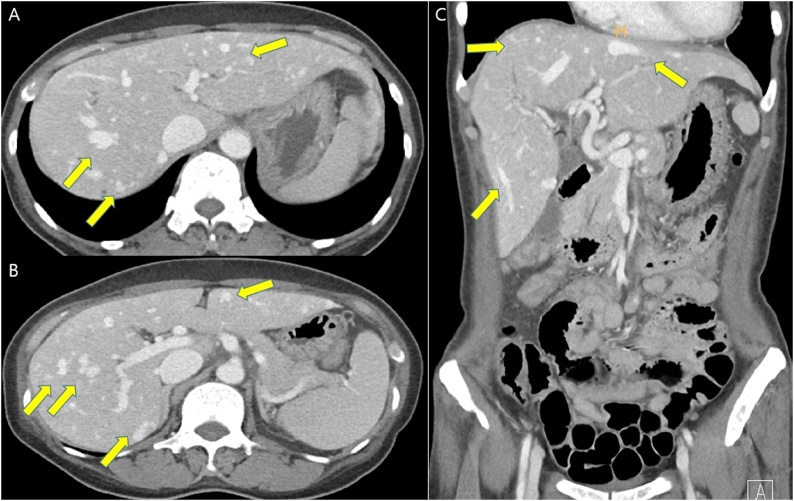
Abdominopelvic CT scan: A & B) Dilated tortouous hepatic arteries with mutifocal arterio-venous shunts in liver, arterio-venous malformation in ileal wall. C) Coronal view.
